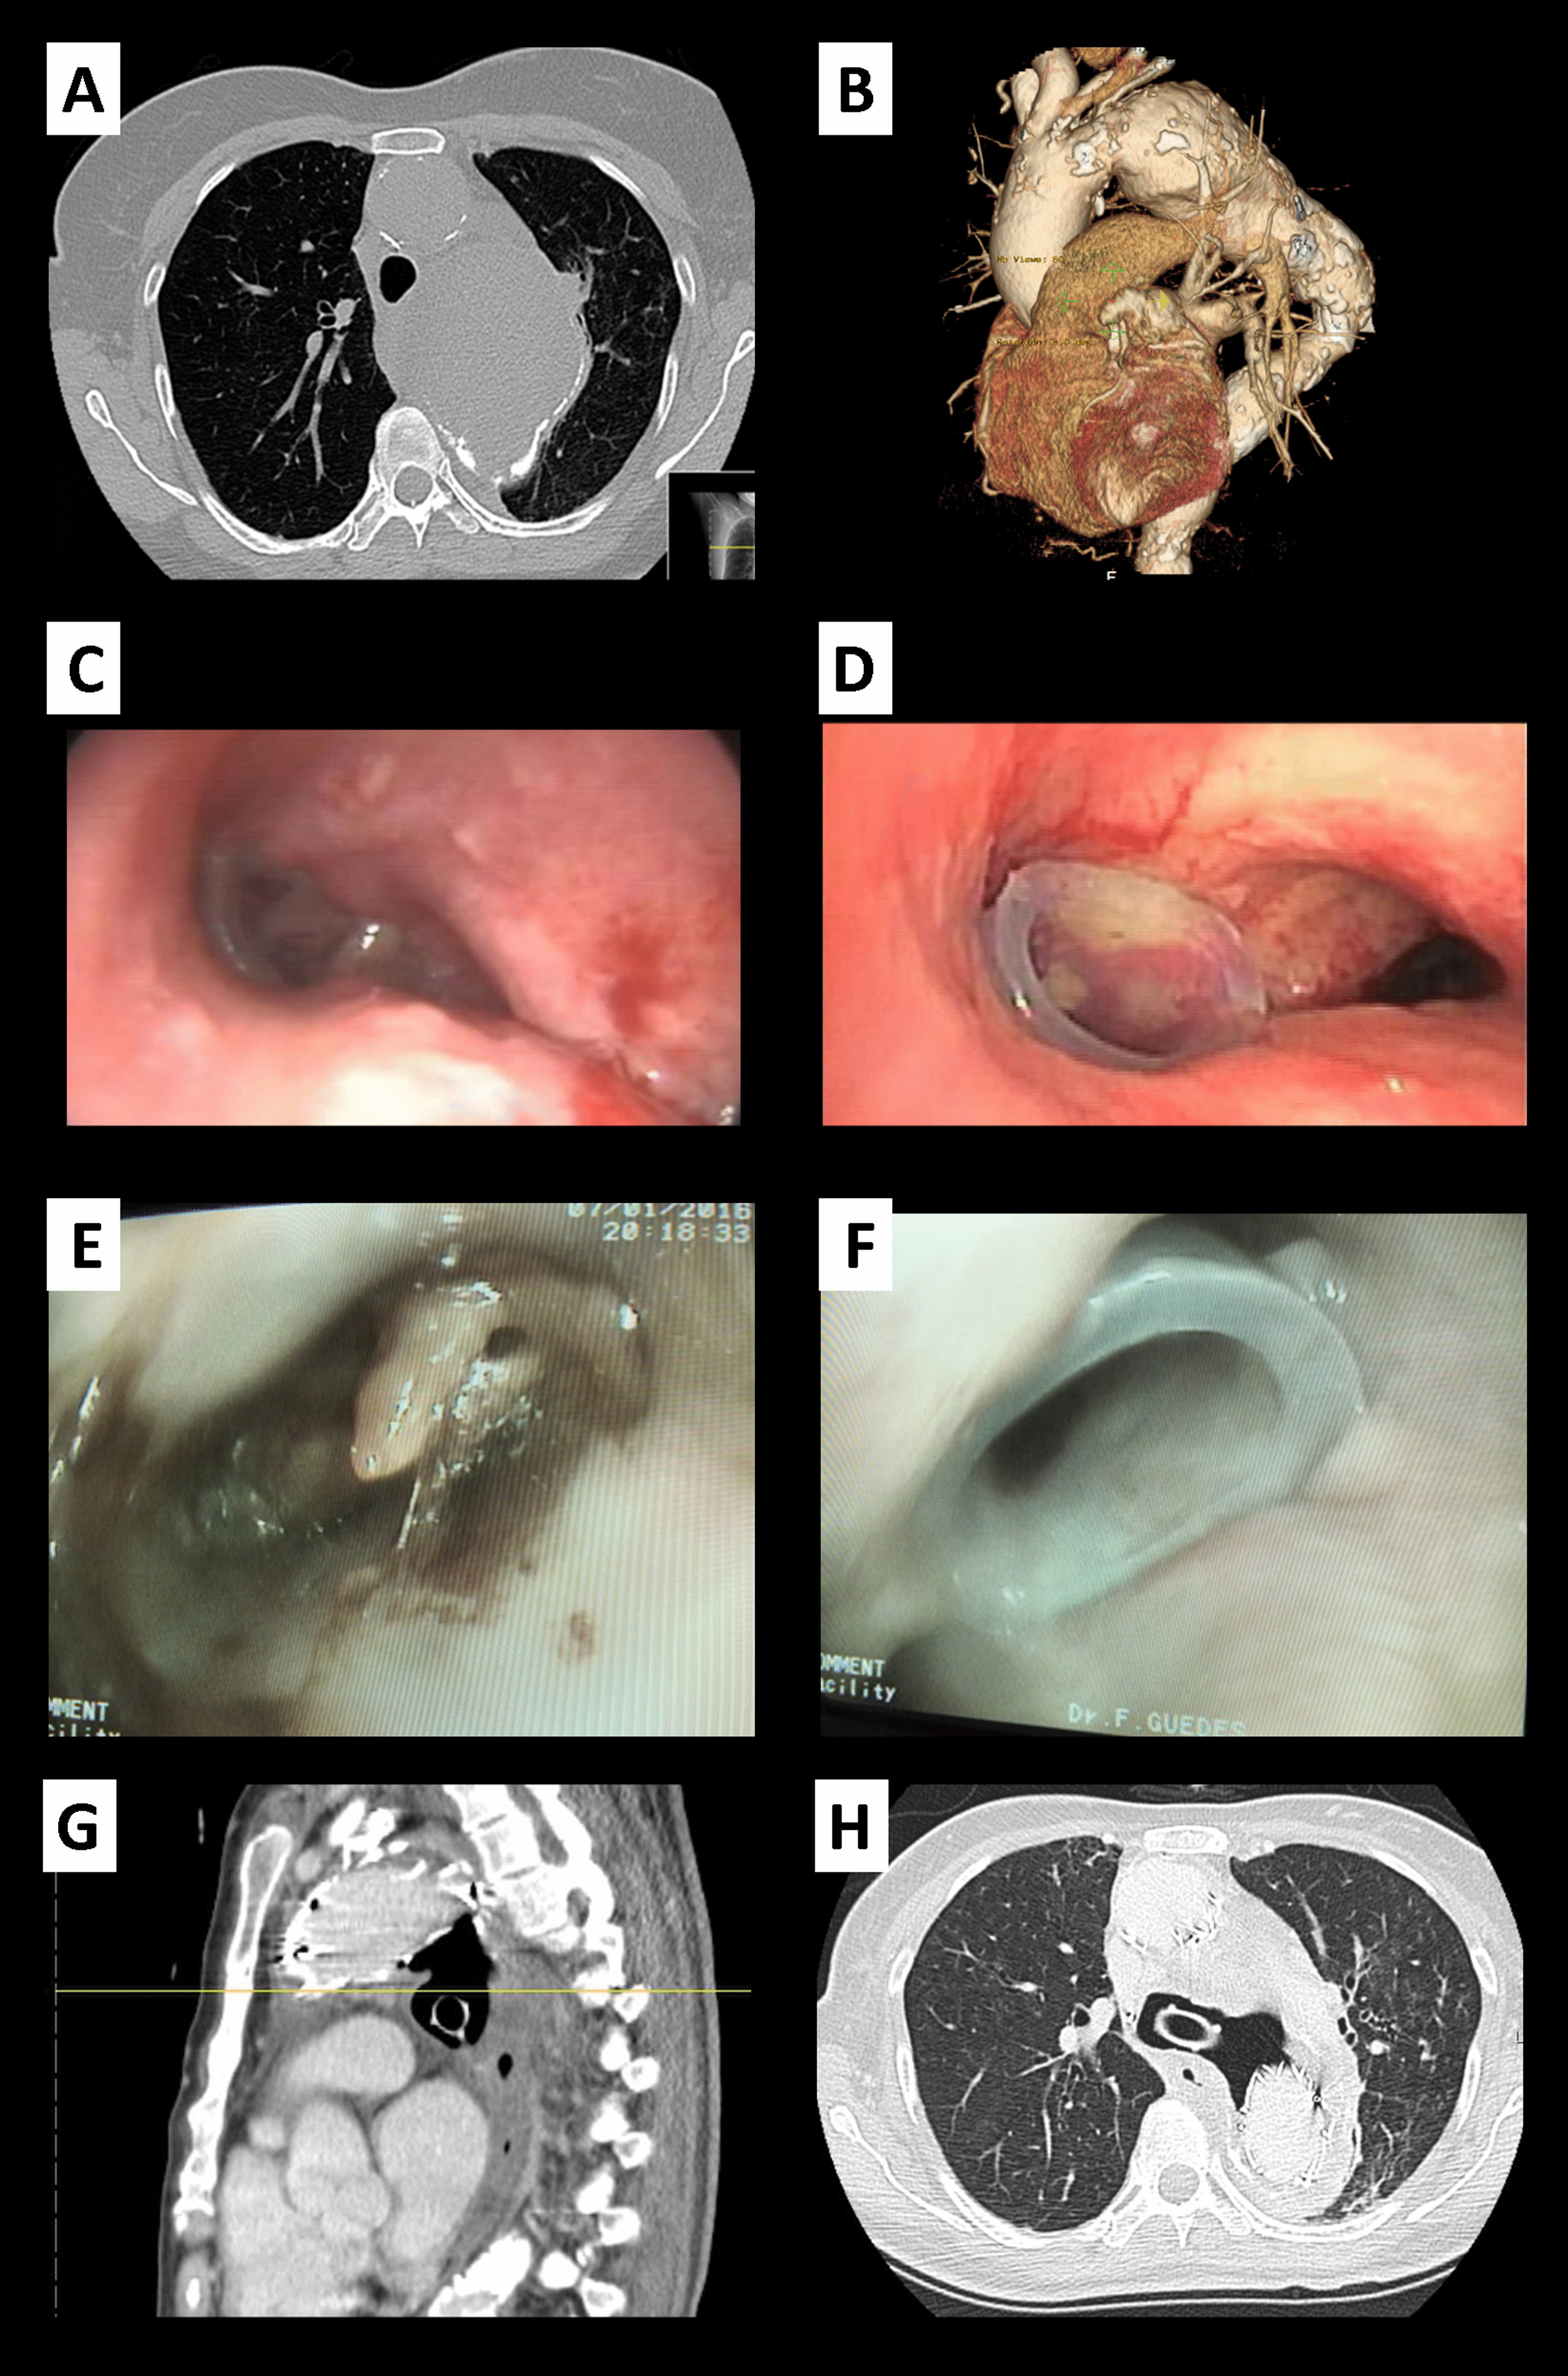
Sequence of happenings observed in thoracic CT scan and in bronchoscopy: A thoracic aorta aneurism with tracheal contact; B 3-D reconstruction of thoracic aorta aneurism; C carina with signs of extrinsic compression; D stent in left main bronchus; E total occlusion of stent witch mucus; F patency of stent post laborious cleaning; G, F evidence of fistulization from LMB to mediastinum, contacting directly to aneurismatic sac
